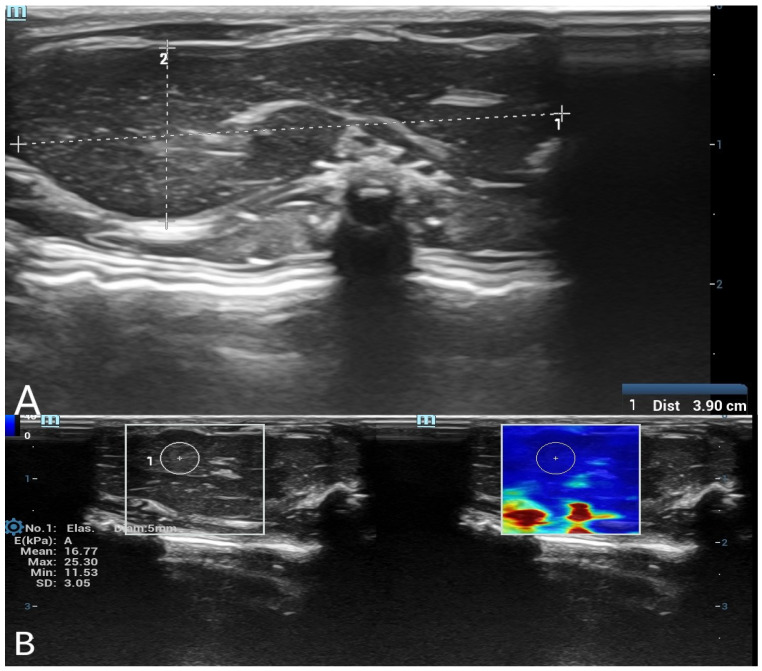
(A) B-mode ultrasound measurement of liver long and short diameters. A grayscale ultrasound image showing the measurement of liver long and short diameters. The calipers indicate the dimensions of the liver in the postmortem assessment. (B) Shear wave elastography (SWE) measurement of liver stiffness with a 5 mm region of interest (ROI). The left panel displays the grayscale ultrasound image of the liver, while the right panel shows the corresponding SWE color map. A 5 mm circular ROI is placed in the liver parenchyma to obtain quantitative stiffness values in kilopascals (kPa).
